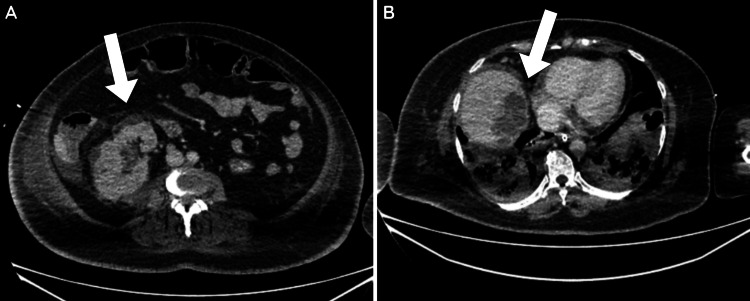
Computed tomography (CT) scan of the abdomen shows abscesses in the hepatic dome and right perinephric region. Figure A shows the right perinephric abscess, and Figure B shows the liver abscess, with arrows pointing to the respective abscesses.
